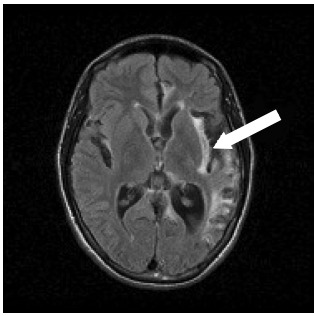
Magnetic resonance imaging showing areas of encephalomalacia with principal involvement of the left temporal lobe, and lesser involvement of the left frontal lobe. These represent areas typically affected by herpes simplex virus.
